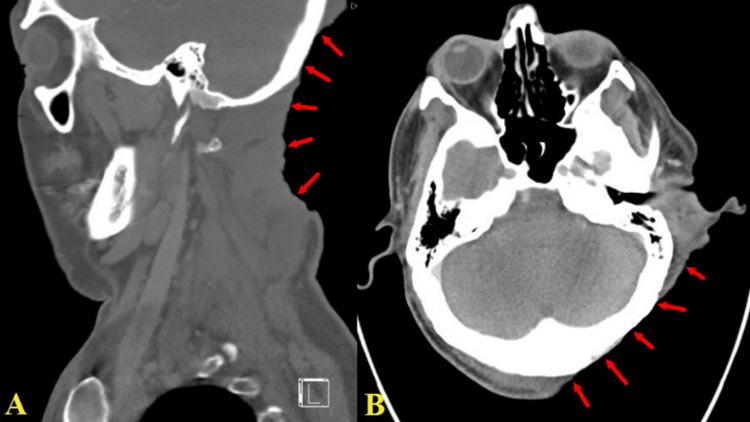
Large skin defect with occipital bone exposure Lateral head and neck (A) and brain (B) CT scan showing deep skin defect on the posterior neck reaching the occipital bones (red arrows). CT: computed tomography
